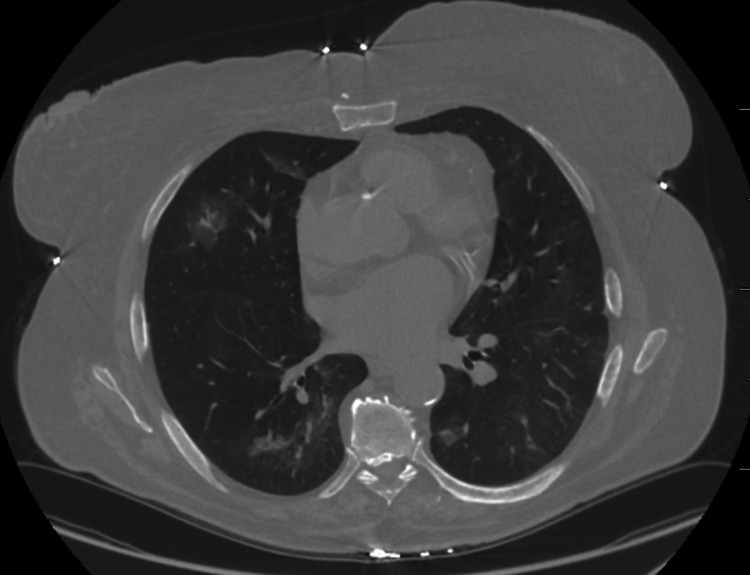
Follow-up CT scan with improvement of previously seen infiltrates after initiation of chemotherapy
